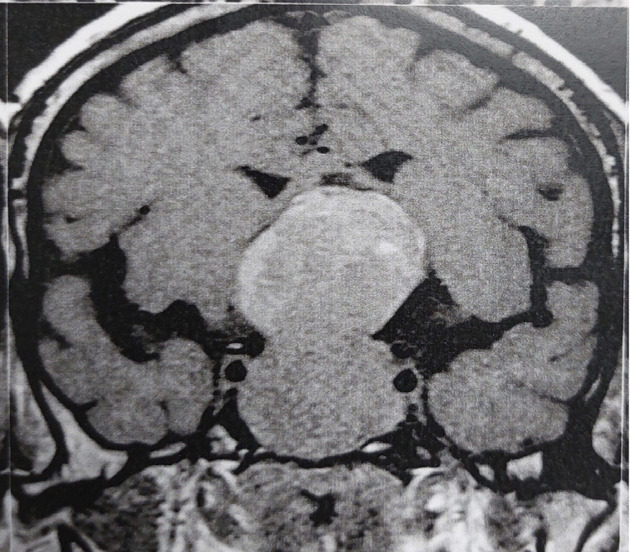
Coronal magnetic resonance imaging of the pituitary showing a giant prolactinoma (6.4 cm) in a patient with falsely low serum prolactin level (91 ng/mL) due to the high-dose hook effect. The prolactin level increased to 22,600 ng/mL after a 1:100 dilution of the serum sample.
